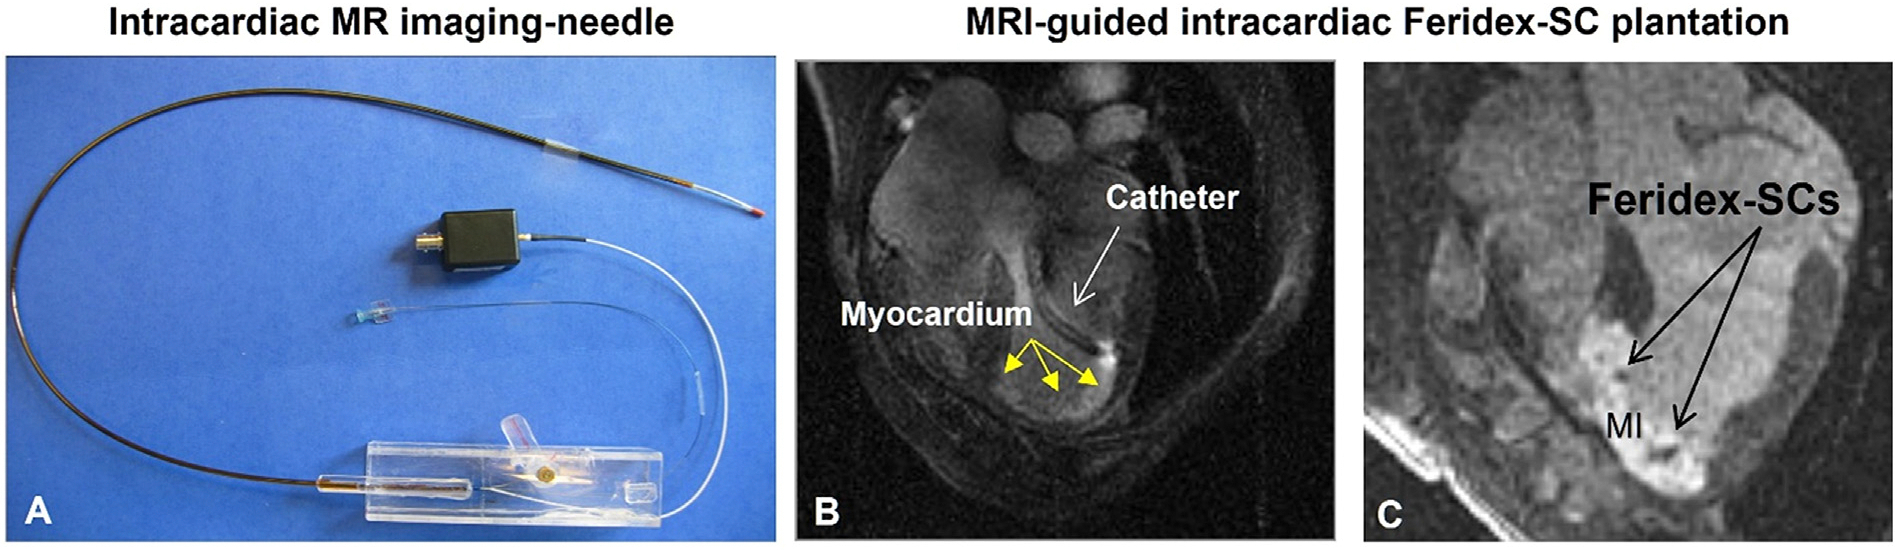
MRI-guided intracardiac stem cell (SC) injection for myocardial ischemia (MI) treatment. (A&B) An active MRI-compatible catheter for intracardiac injection of therapeutic agents or stem cells into the myocardium under MR guidance (Courtesy of Parag Karmarkar). (C) Long-axis MRI showing a hypointense SC cluster (arrows), resulting from SCs labeled by a T2-MR contrast agent, Feridex (Courtesy of Ergin Atalar).
